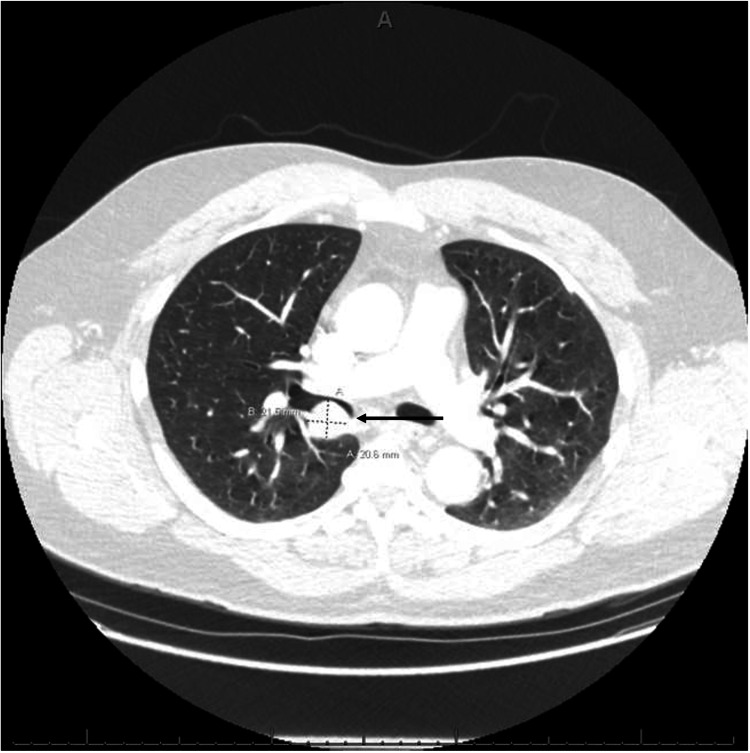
Transverse section of the chest CT showing the endobronchial mass (black arrow). CT: computed tomography
